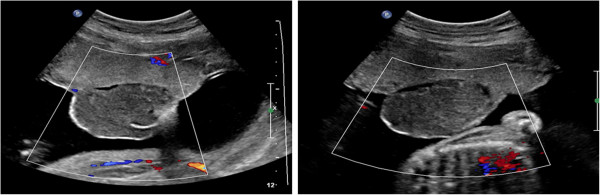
Chorioangioma post embolization. Ultrasound images showing no flow through the tumor.
